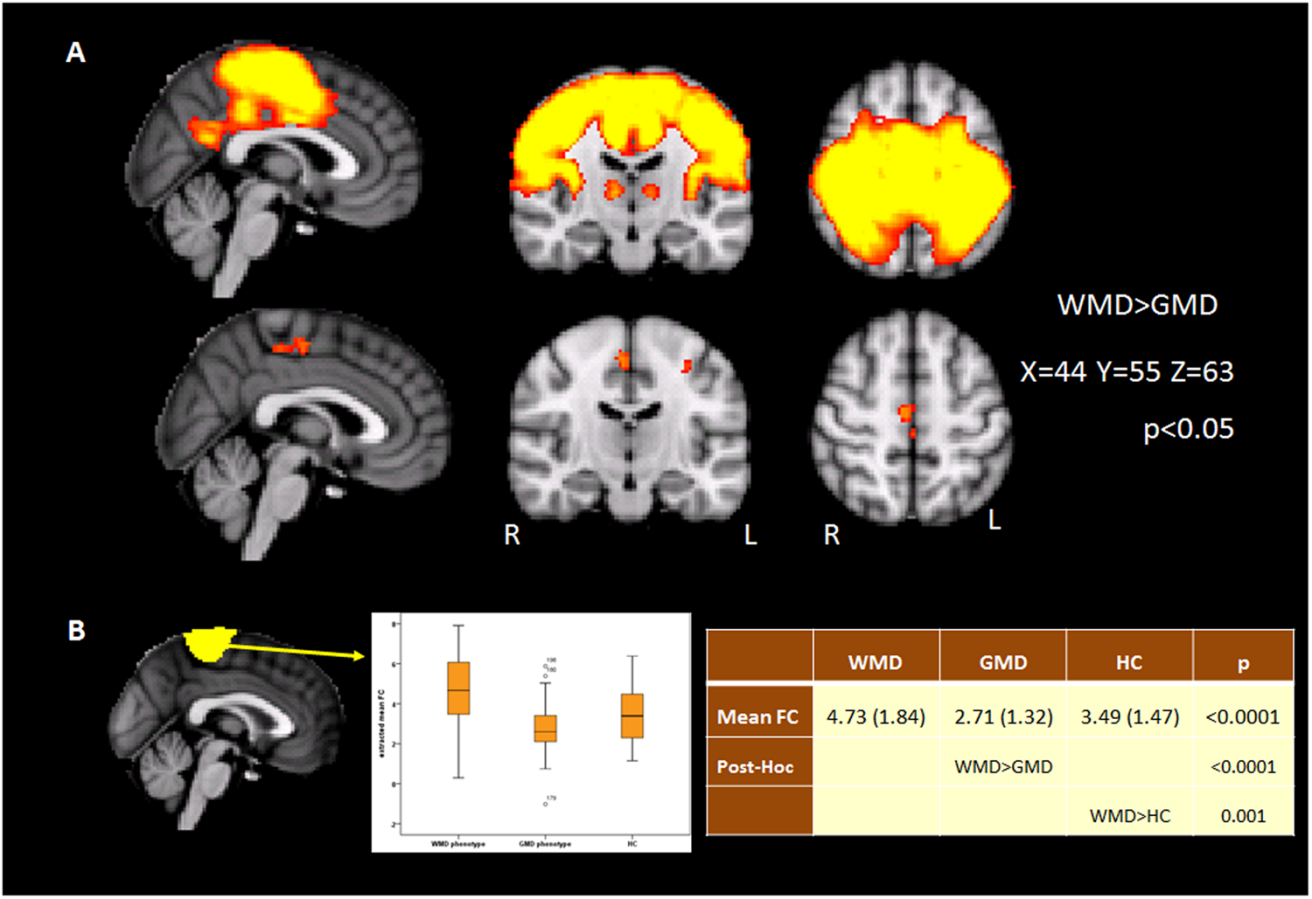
(A) Increased FC in the sensorimotor network in patients with the primarily white (WMD) compared to the grey matter damage (GMD) MRI-phenotype. (B) Increased mean FC in the central sub-unit (leg area) in WMD patients compared to GMD and HC.
